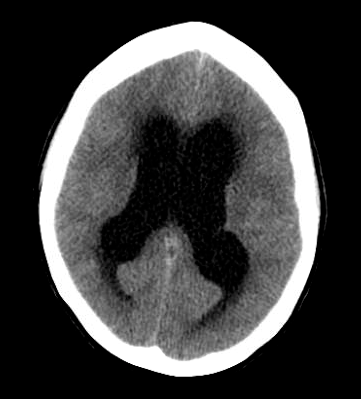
CT of the Brain without Contrast A: Axial image demonstrating dilated lateral ventricles with transependymal edema
